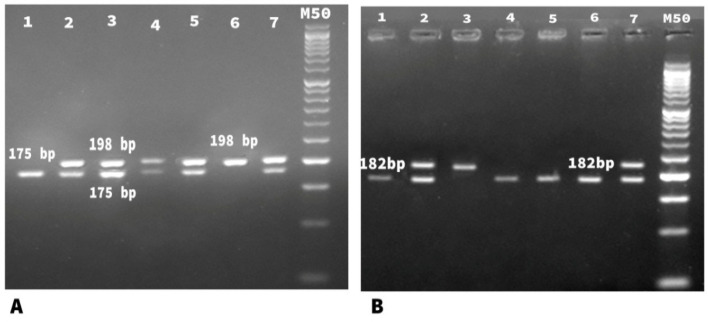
Agarose Gel Electrophoresis of Digested MTHFR C677T & MTHFR A1289C Amplicons (A) *MTHFR C677T*: Lane 1represents homozygous cut (TT) (175bp), Lanes 2,3,4,5,7 represent heterozygous cut (CT) (198bp & 175 bp), Lane 6 shows no cut (CC) (198bp), (M) represents 50bp molecular weight marker. (B) *MTHFR A1289C*: Lanes 1,4,5,6 show homozygous cut (CC) (182bp), Lanes 2,7 show heterozygous cut (AC) (237 bp and 182 bp), Lane 3 represents no cut (AA) .(M) represents 50bp molecular weight marker
